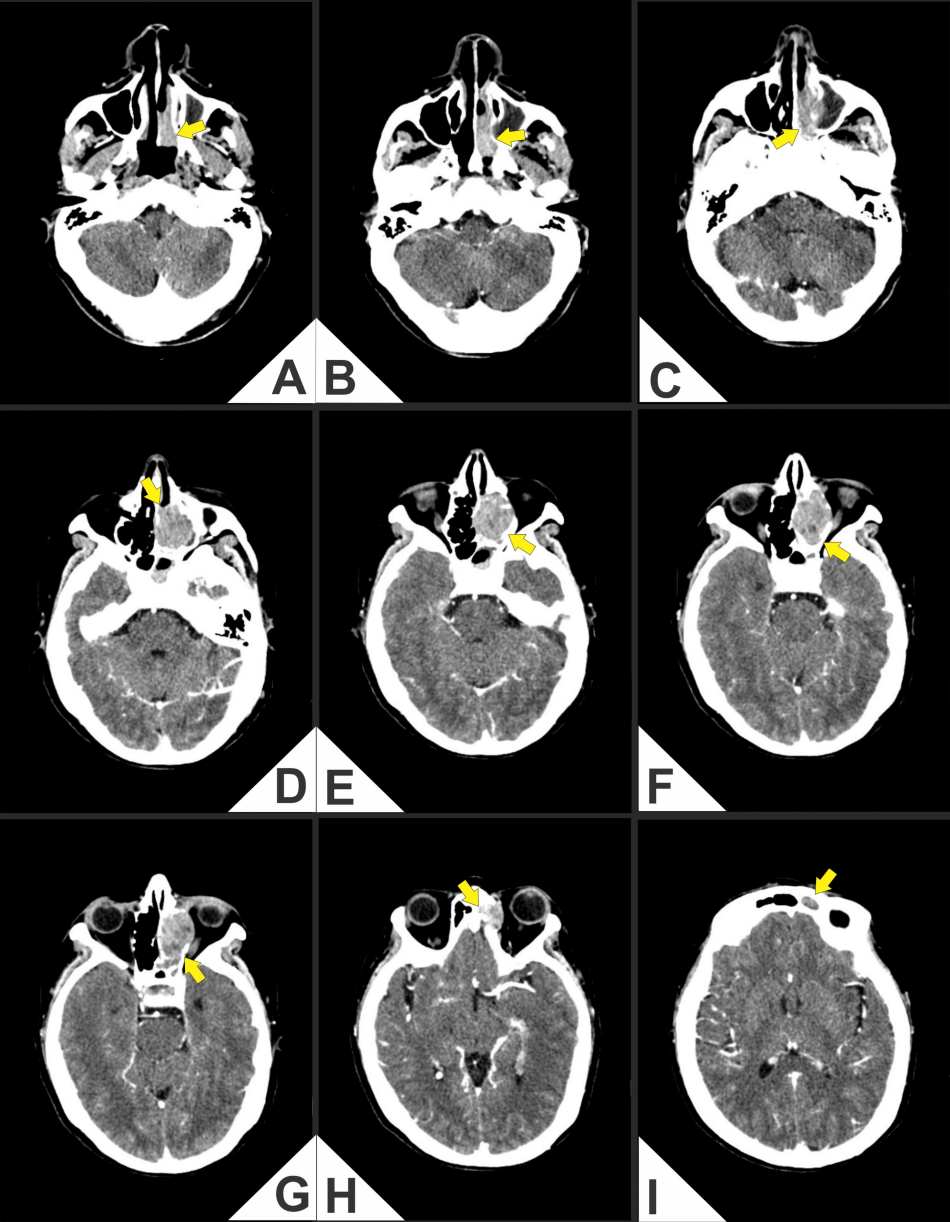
(A-I) Contrast-enhanced axial computed tomography (CT) of the paranasal sinuses and anterior skull base. Heterogeneously enhancing soft-tissue mass centered in the left nasoethmoid-maxillary complex (arrows) with hypodense areas, causing remodeling and erosion of the lamina papyracea and extension to the medial orbit with contact and displacement of the medial rectus muscle, accompanied by effacement of the extraconal fat plane and no definite intraconal involvement (D-G). On more cranial sections (H, I), cortical irregularity and probable dehiscence of the ethmoid roof/cribriform plate region are identified, suggesting anterior skull base involvement. Reactive opacification of ethmoid air cells and adjacent paranasal sinuses is present.
